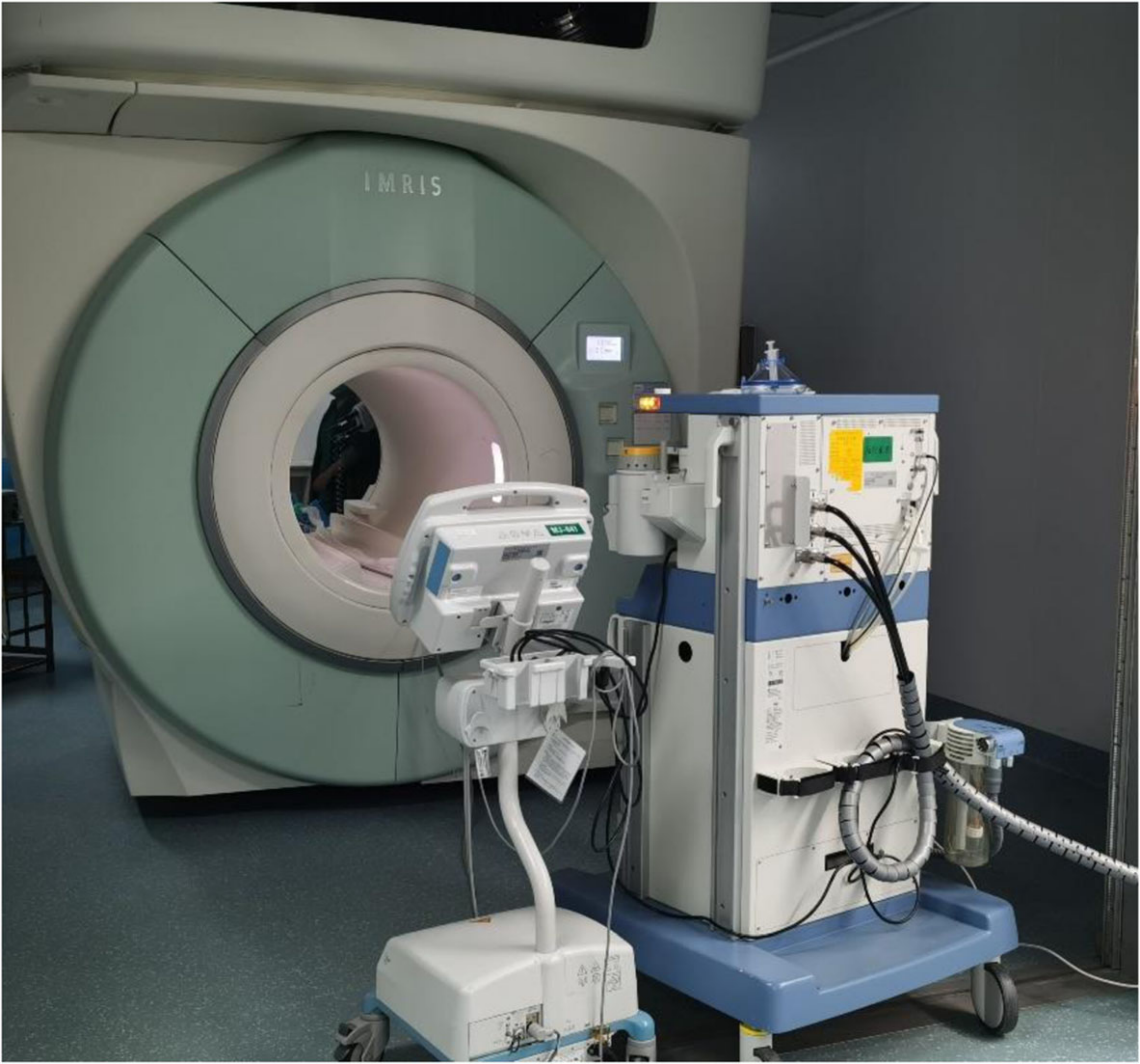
Magnetic resonance imaging and placement of anesthesia equipment during treatment
